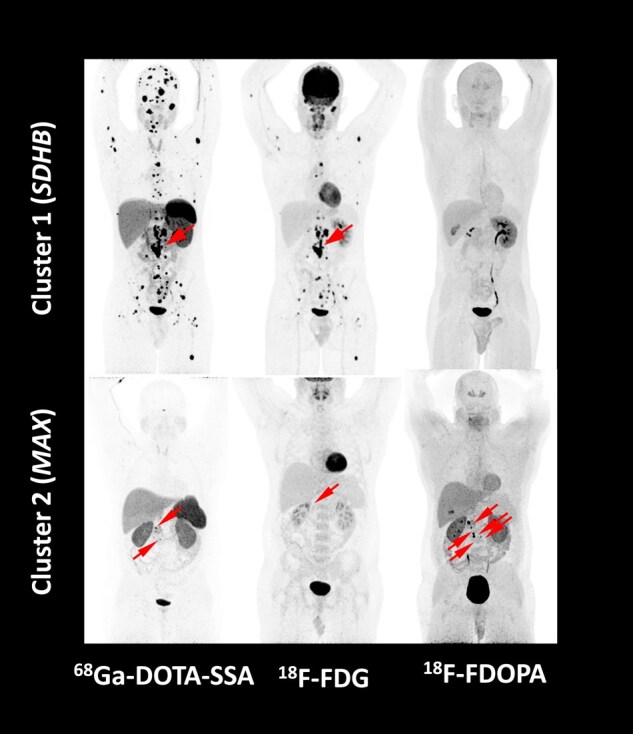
Impact of genetic cluster on functional imaging. PET images of a 20-year-old male with cluster 1 PPGL related to an *SDHB* pathogenic variant (upper panels) and of a 38-year-old male with PCC related to a cluster 2 *MAX* pathogenic variant. Abbreviations: FDG, fluorodeoxyglucose; FDOPA, fluorodihydroxyphenylalanine; *MAX*, MYC-associated factor X; *SDHB*, succinate dehydrogenase B; SSA, somatostatin analogue.
